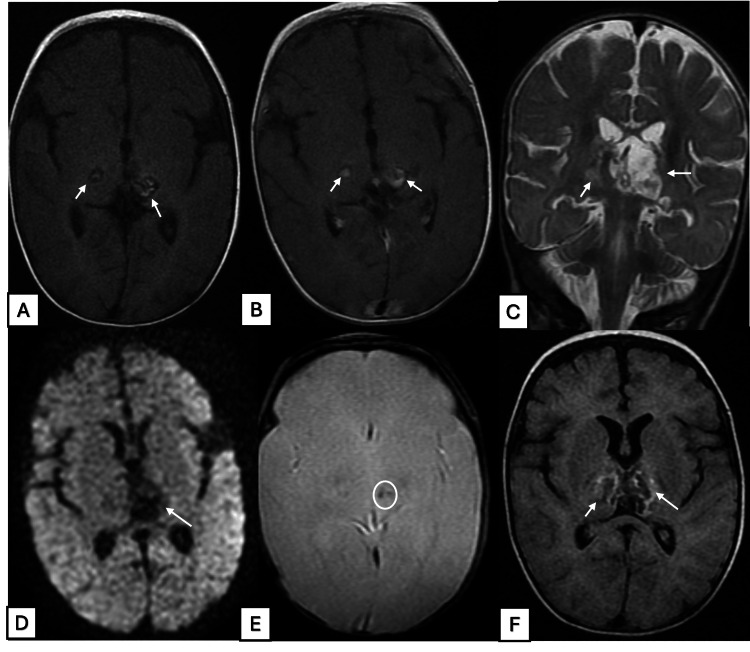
Multimodal MRI findings in ANEC Axial FLAIR and T1-weighted images (A, B) and coronal T2-weighted images (C) show poorly defined hyperintense lesions in the thalami, containing focal areas of hyperintensity (arrows). Axial DWI (D) shows little to no diffusion restriction. T2* gradient-echo imaging (E) demonstrates areas of blooming artifact consistent with hemorrhage (arrowheads). Post-contrast axial T1-weighted images (F) show central annular and heterogeneous enhancement. ANEC, acute necrotizing encephalopathy of childhood; DWI, diffusion-weighted imaging
